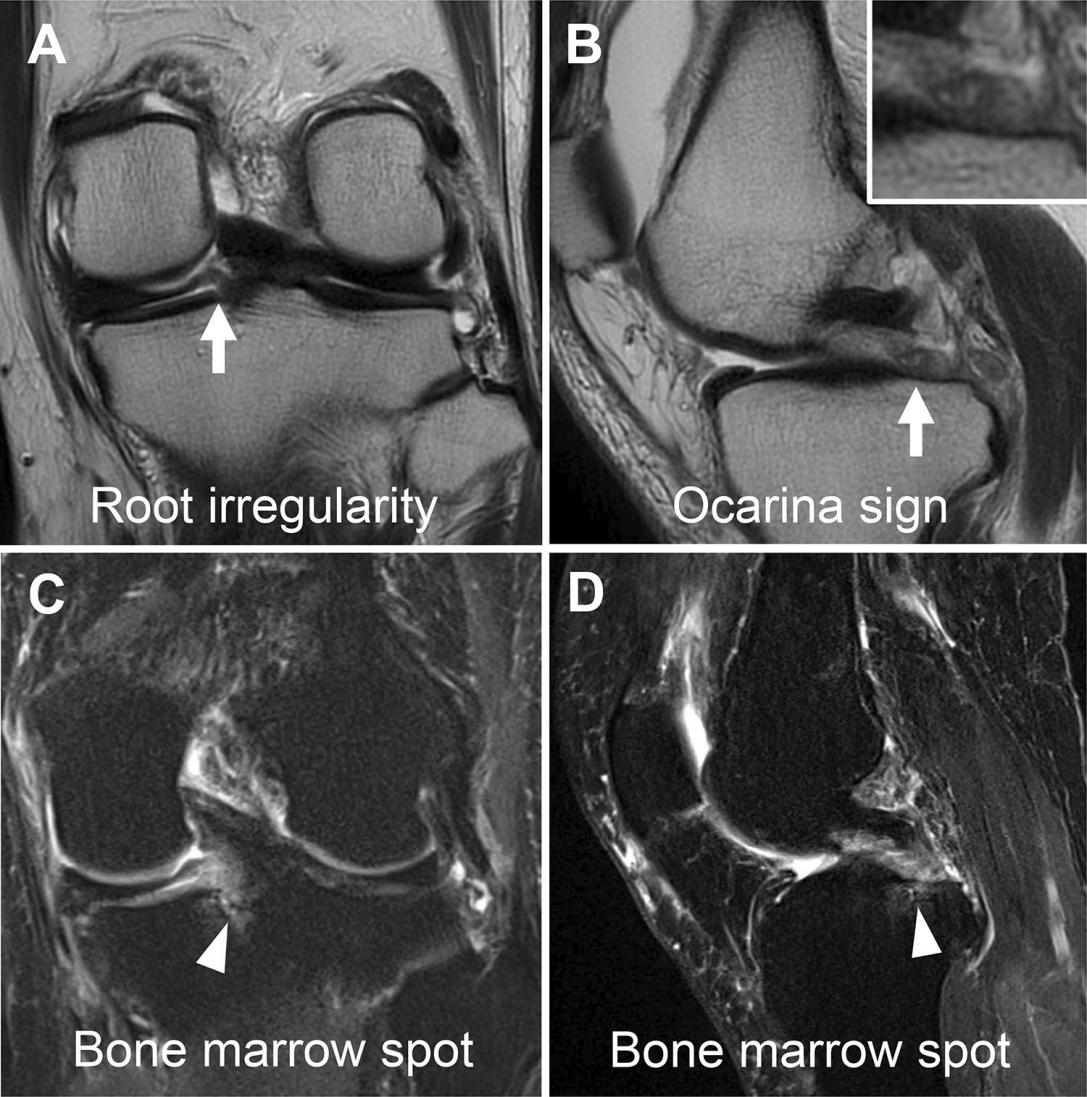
MRI findings of partial MMPRTs. Partial MMPRTs diagnosed via MRI. a Root irregularity (arrow, coronal T2-weighted image). b Ocarina sign (arrow, sagittal T2-weighted image). c Bone marrow spot (arrowhead, coronal T2-weighted fat-suppressed image). d Bone marrow spot (arrowhead, sagittal T2-weighted fat-suppressed image). *MMPRTs* medial meniscus posterior root tears, *MRI* magnetic resonance imaging
